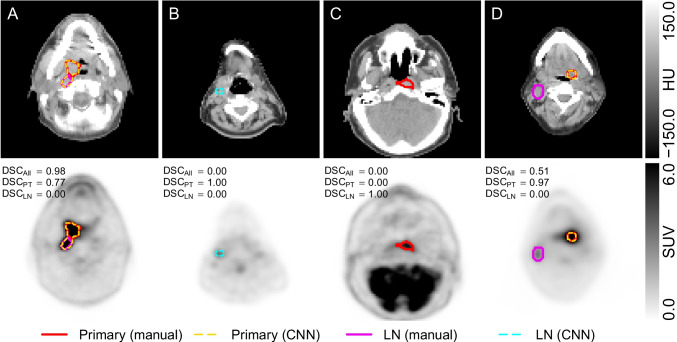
Examples of CNN delineation errors in 4 selected patients. Relevant transaxial PET/CT slices are shown (top: CT, bottom: PET). The dice coefficients (in the presented plane) for primary tumor ($$\text {DSC}_{\text {PT}}$$), LN metastases ($$\text {DSC}_{\text {LN}}$$) and their union ($$\text {DSC}_{\text {All}}$$) are indicated. Patient A with laryngeal cancer and multiple LN metastases (only one in plane): LN metastasis incorrectly classified as primary; patient B with oropharyngeal cancer and LN metastasis (both out of plane): CNN produced spurious LN metastasis ROI; patient C with nasopharyngeal cancer and LN metastases (out of plane): primary missed by CNN; patient D with oropharyngeal cancer and low and diffuse uptake LN metastasis: LN metastasis missed by CNN
